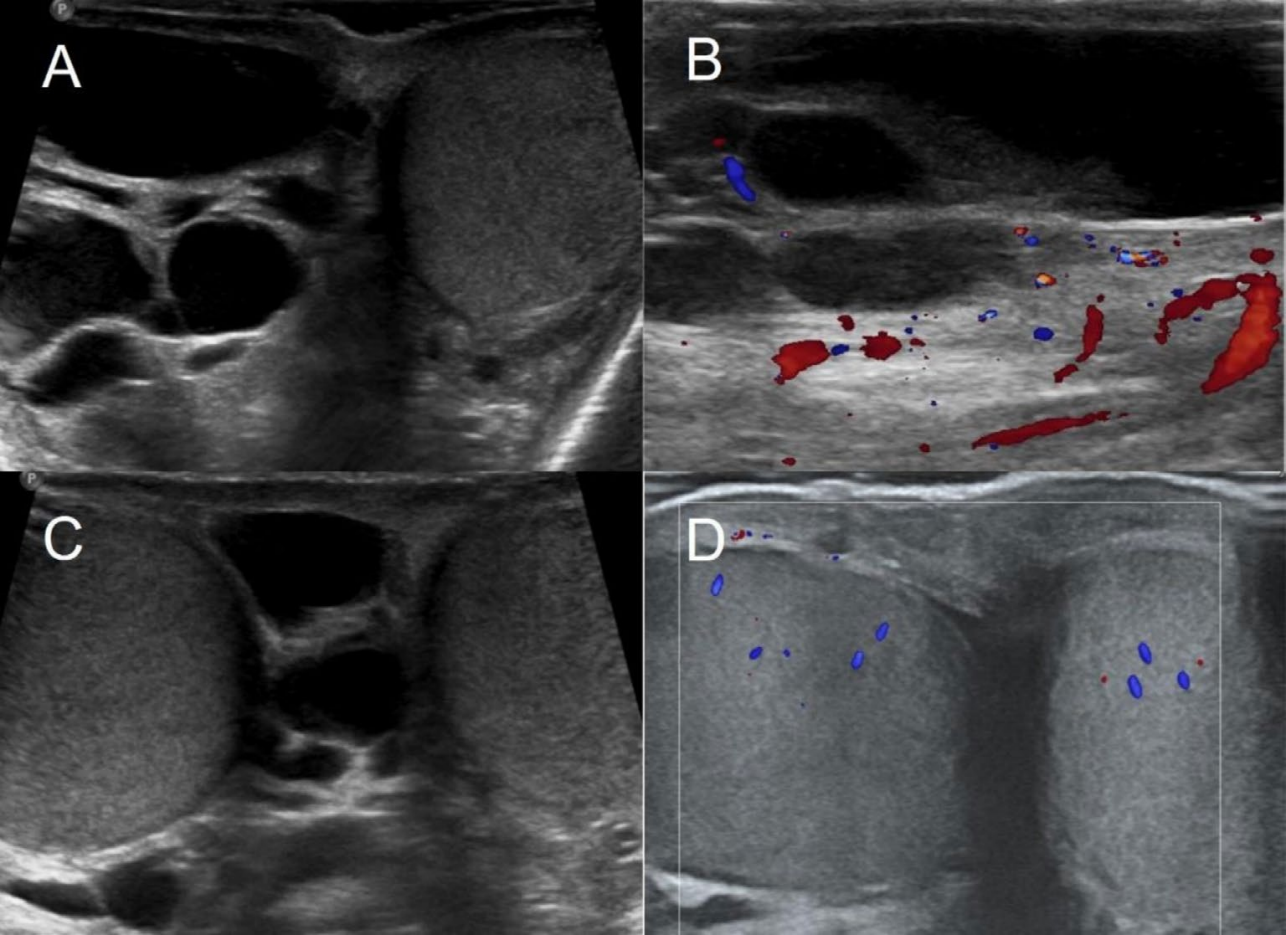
Ultrasound examination. (A–C) Are the preoperative color ultrasound images. (D) Shows the color ultrasound image obtained during the one‐year postoperative follow‐up.
